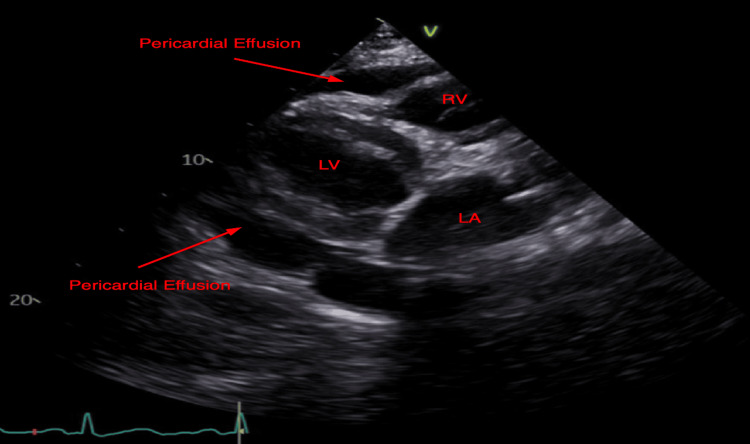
Parasternal long-axis (PSLA) echocardiogram - Moderate-to-large circumferential pericardial effusion with right ventricular collapse RV, right ventricle; LV, left ventricle; LA, left atrium.
